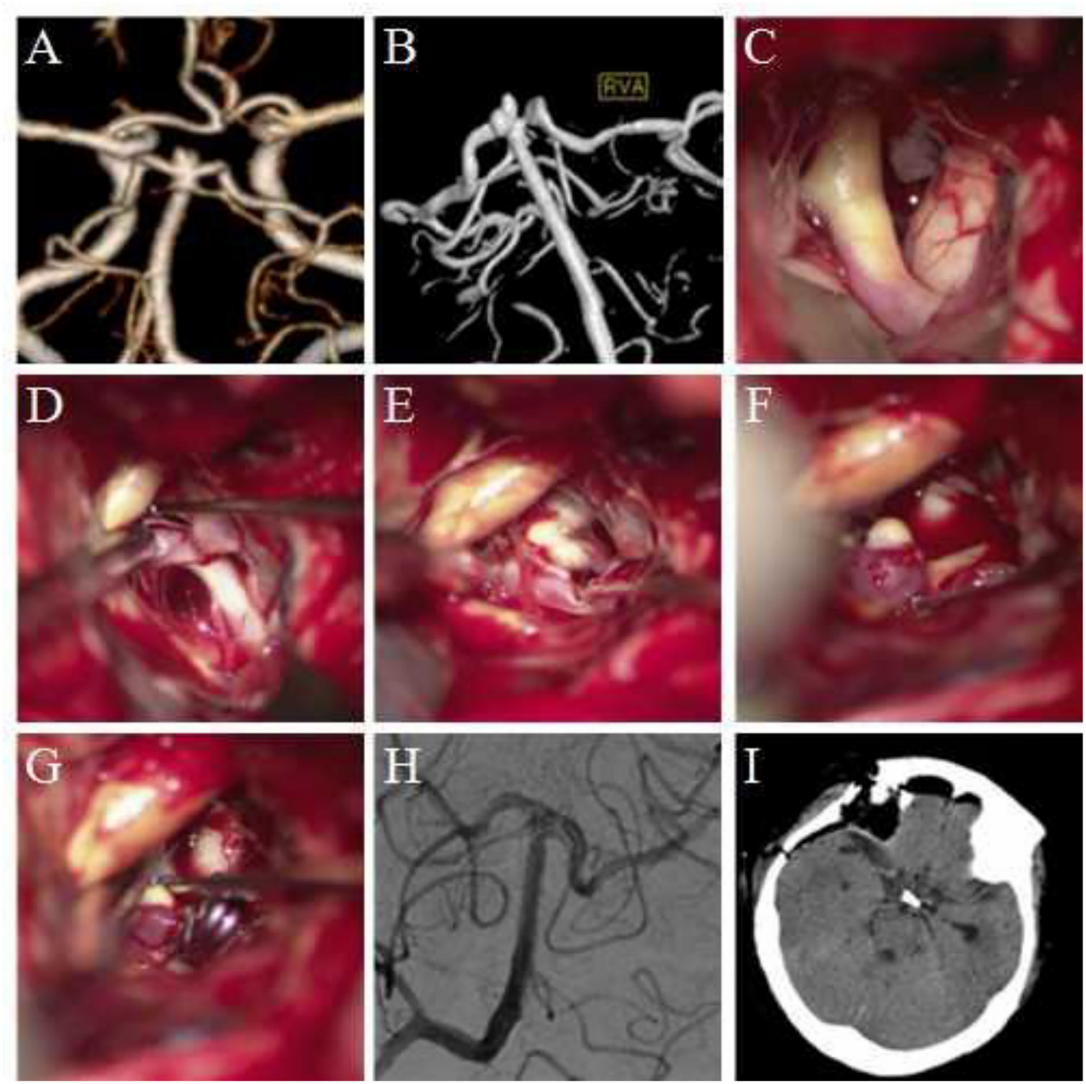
Clipping of basilar artery apical aneurysm *via* the Dolenc approach. (A,B) Preoperative computed tomography angiography (CTA) and digital subtraction angiography (DSA) revealed a basilar artery apical aneurysm; the aneurysm was lobulated. (C) During surgery, the internal carotid artery and the posterior clinoid process of the surgery side were exposed. (D) The internal carotid artery was pulled medially, and the exposure range was increased. The P1 segment of the posterior cerebral artery and its perforating arteries were visible. (E) The basilar artery was exposed along the posterior cerebral artery to the top of the basilar artery. The aneurysm was exposed, and the sub-aneurysm was visible. (F) The aneurysm was completely exposed, and sub-aneurysms were visible. (G) The neck of the aneurysm was clipped. The basilar arteries, bilateral posterior cerebral arteries, and superior cerebellar arteries were explored, and no vascular damage was observed. (H) Intraoperative DSA revealed no development of aneurysm and no vascular damage. (I) Re-examination was performed using the head CT within 12 h after surgery.
